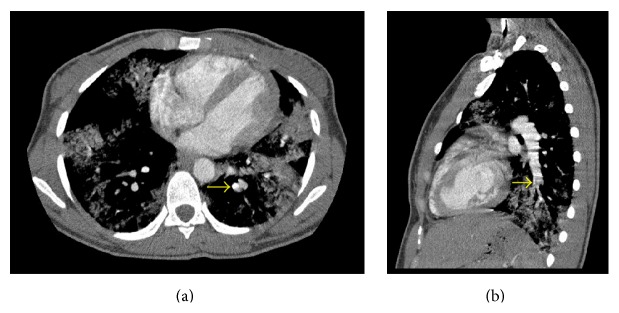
Volumetric computed tomography acquisition of the chest from the thoracic inlet to the upper abdomen, following 65 mL of intravenous contrast (Omnipaque 300) employing pulmonary embolism protocol. A filling defect has been demonstrated in the left lower lobe segmental pulmonary artery (arrows: (a) axial; (b) sagittal).
